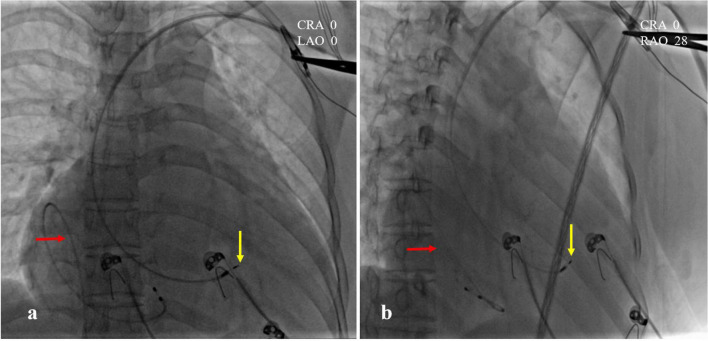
Intraoperative X-ray fluoroscopy images. (a) CRA 0, LAO 0; (b) CRA 0, RAO 28. The temporary pacemaker lead was coiled in the right atrial lumen (red arrow). The position of left bundle branch area pacing (LBBaP) was at the distal end of the ventricle, 1.5 cm approximately beyond HIS (yellow arrow).
